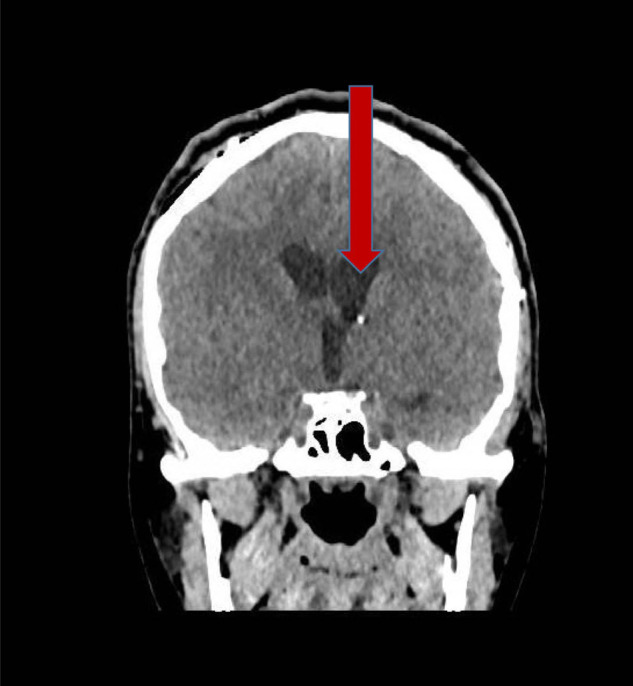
A computed tomography post-external ventricular drain (day 4) image showing resolved tension pneumoventricles (red arrow)
